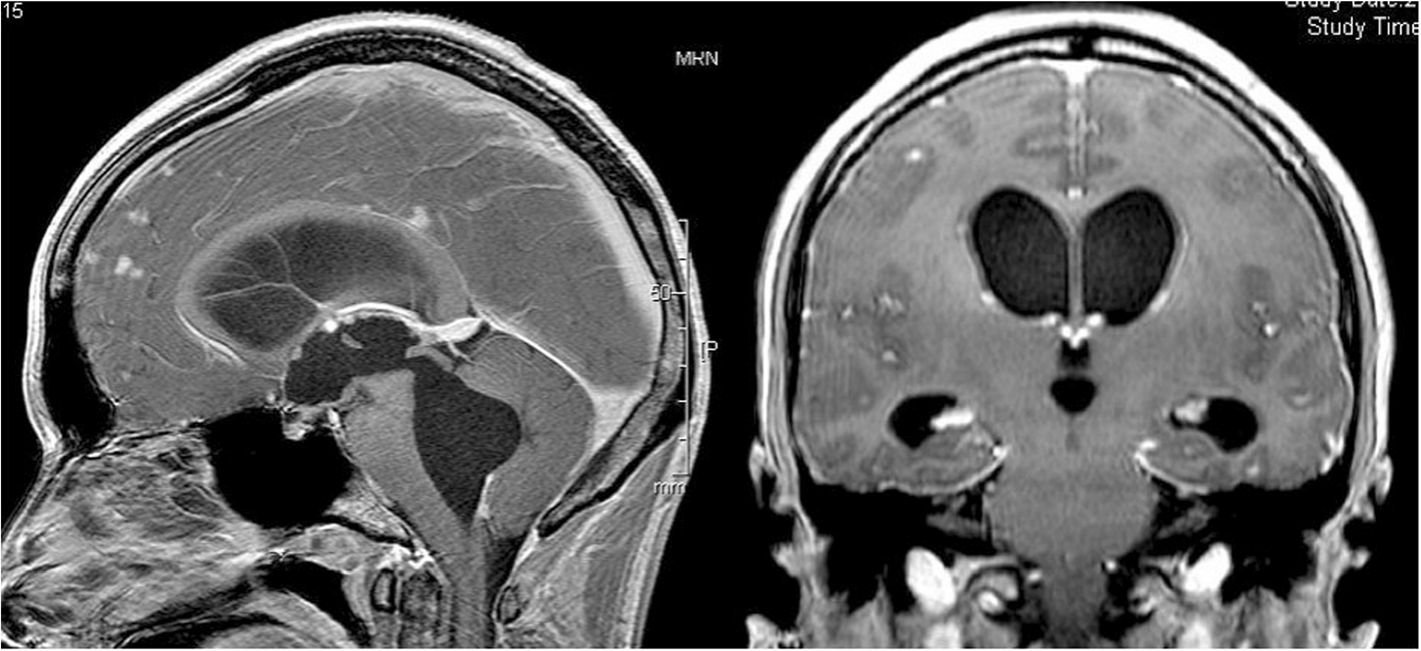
Preoperative contrast-enhanced magnetic resonance imaging showed millet seed-like enhancement along the third ventricular wall, multiple small enhanced lesions along the Virchow–Robin spaces, and abnormal enhancement near the foramen of Magendie.
